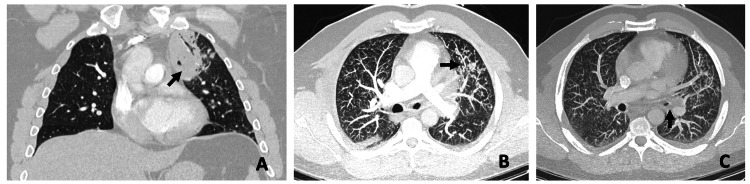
Computed tomography angiography (CTA) images of patient's chest. (A) Mass-like opacity with air bronchograms in medial left upper lung lobe with (B) multiple bronchocentric tree-in-bud nodules in the left upper lobe​ and (C) left hilar lymphadenopathy
